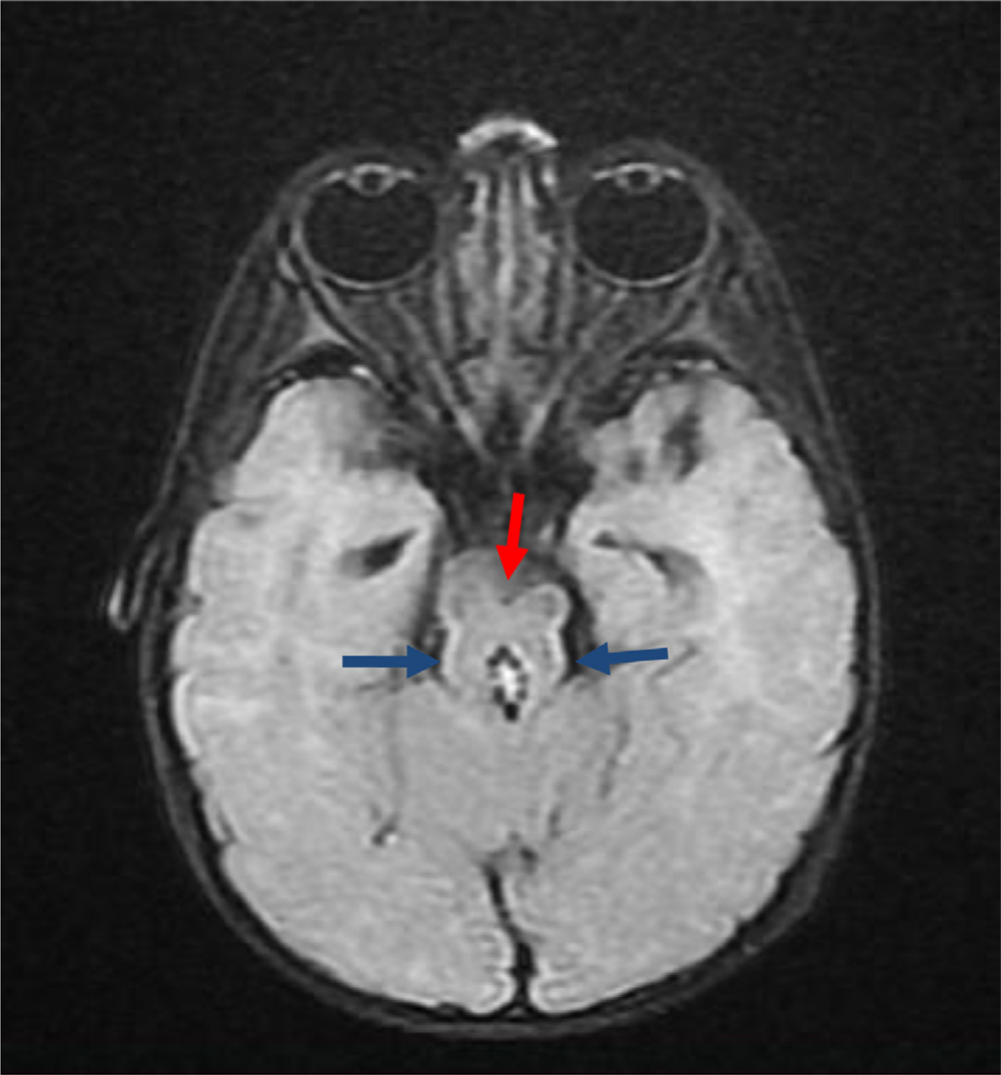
FLAIR sequence—axial section. T1-weighted image (T1WI), T2-weighted image (T2WI), and fluid-attenuated inversion recovery (FLAIR) sequences showing elongated and thickened superior cerebellar peduncles (marked by blue arrow) with hypoplasia of isthmus and deep cleft along anterior midbrain (marked by red arrow) giving characteristic molar tooth sign as marked by white arrows. Interpeduncular fossa is also widened. There is associated hypoplasia of cerebellar vermis.
